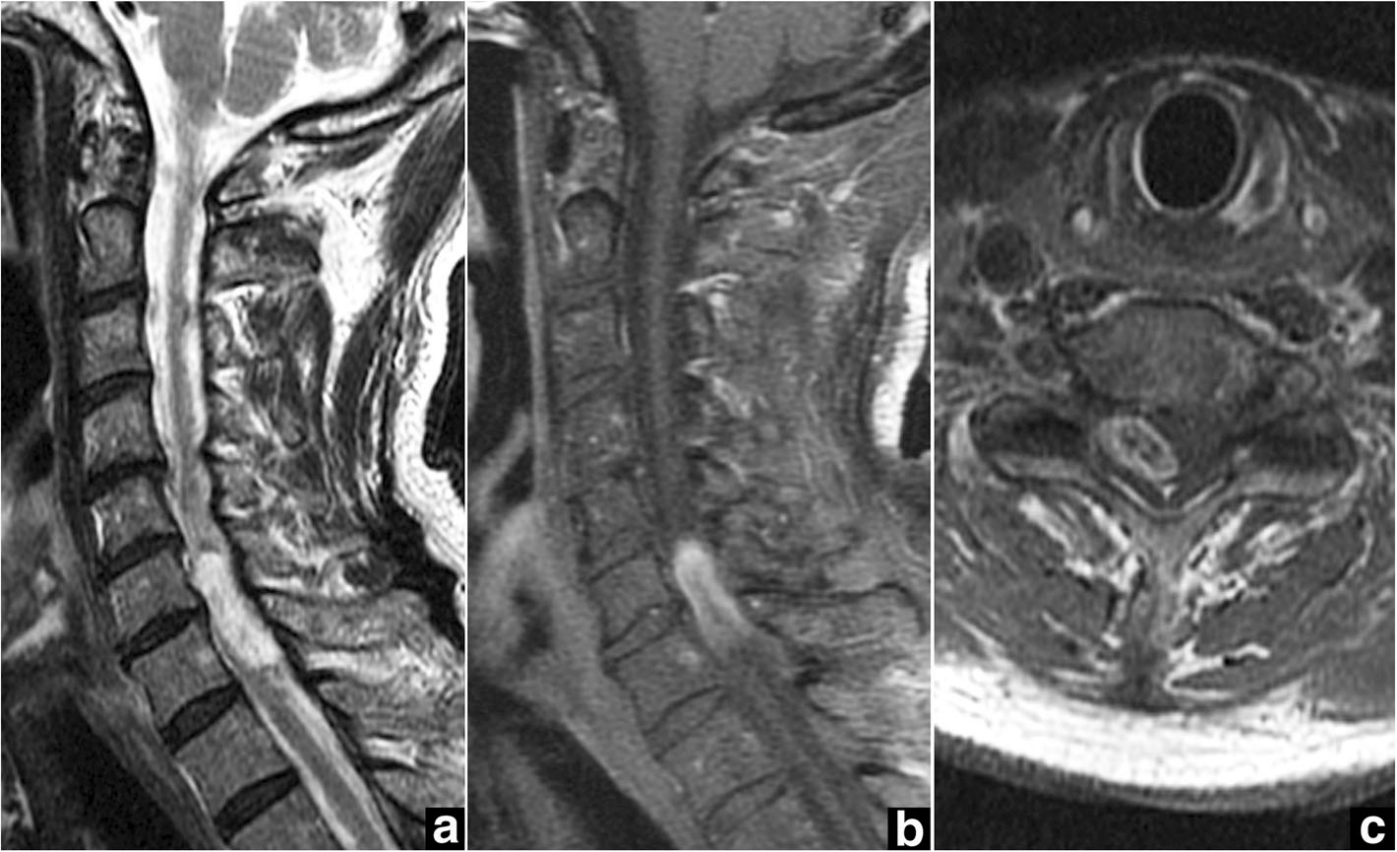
Case 1. Preoperative magnetic resonance imaging (MRI) of the cervical spine. a Sagittal T2-weighted MRI shows the intradural extramedullary spinal cord tumor with signal hyperintensity at C6-C7 and spinal canal stenosis at C4-C7. b, c Gadolinium-enhanced sagittal MRI (b) and axial MRI (c) at the C6-C7 level show a heterogeneously enhanced tumor located dorsally and to the right of the spinal cord
